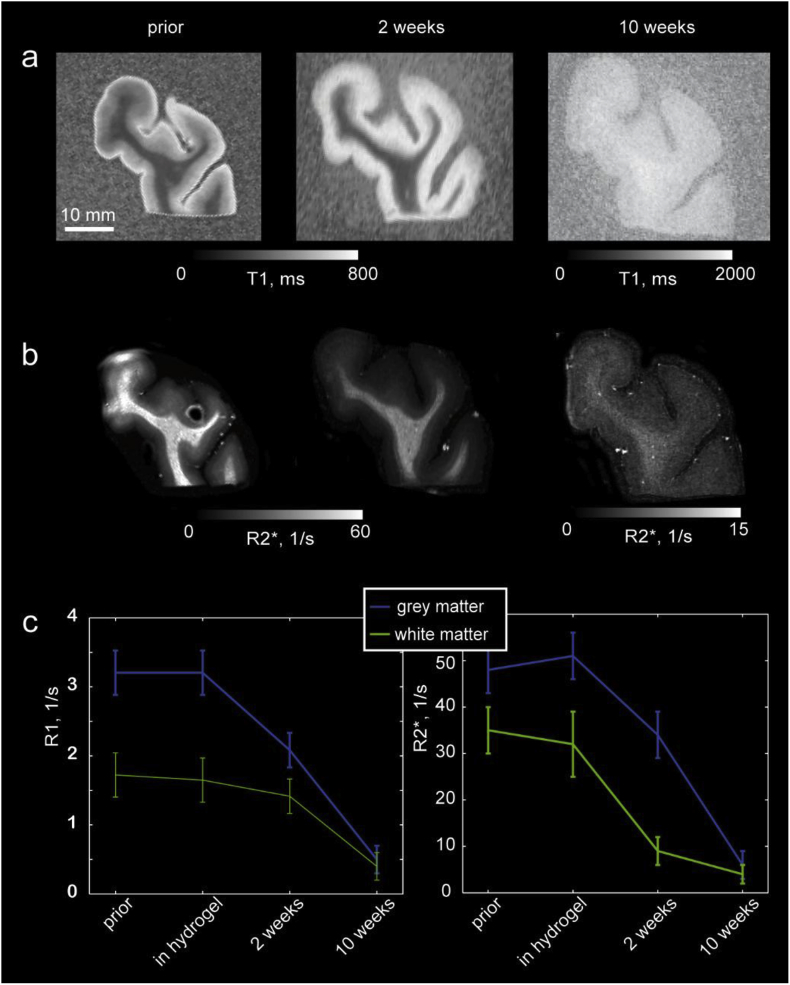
MRI contrast changes upon tissue clearing. Quantitative T1 (a) and R2* maps (b) of a tissue block (Case 1) at three stages of the clearing process (left: prior to clearing, middle: after 2 weeks of clearing, right: after 10 weeks of clearing) for the brain tissue sample from temporal lobe. Note the tissue expansion at later clearing stages. (c) Averaged R1 (=1/T1) and R2* values for grey and white matter at different stages of tissue clearing. MR contrast between grey and white matter disappeared after lipid removal in line with the strong contribution of myelin to T1 and T2* contrasts.
